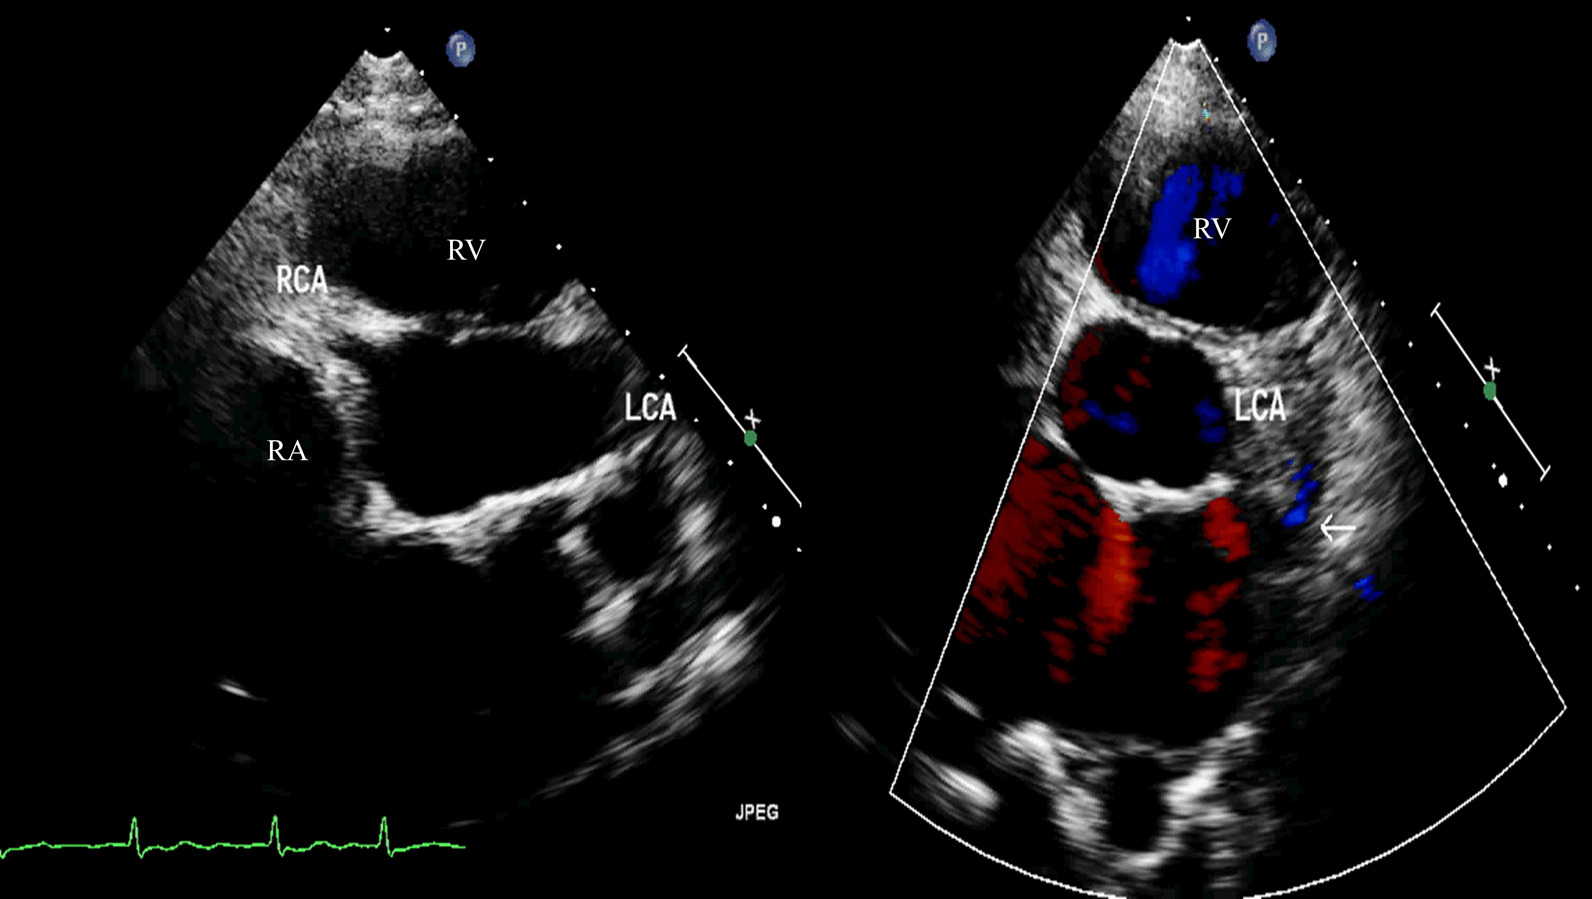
Transthoracic echocardiography showed a giant coronary aneurysm originated from distal left coronary artery and normal right coronary artery (LCA: Left coronary artery; RCA: Right coronary artery; RV: Right ventricle; RA: Right atrium)
